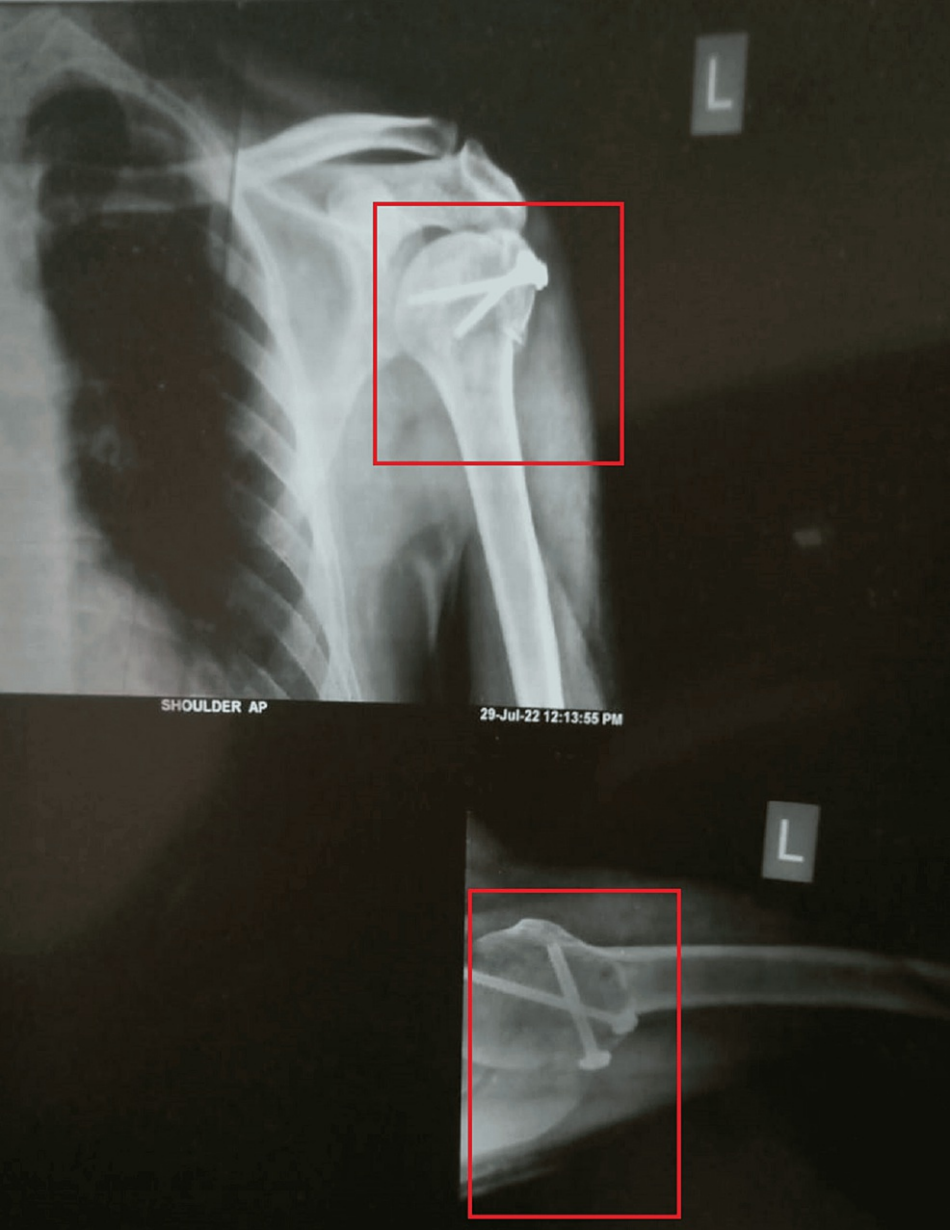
Postoperative anteroposterior X-ray of the left shoulder joint presenting internal fixation with screws for fracture of greater tuberosity of the humerus, reducing the displacement.
